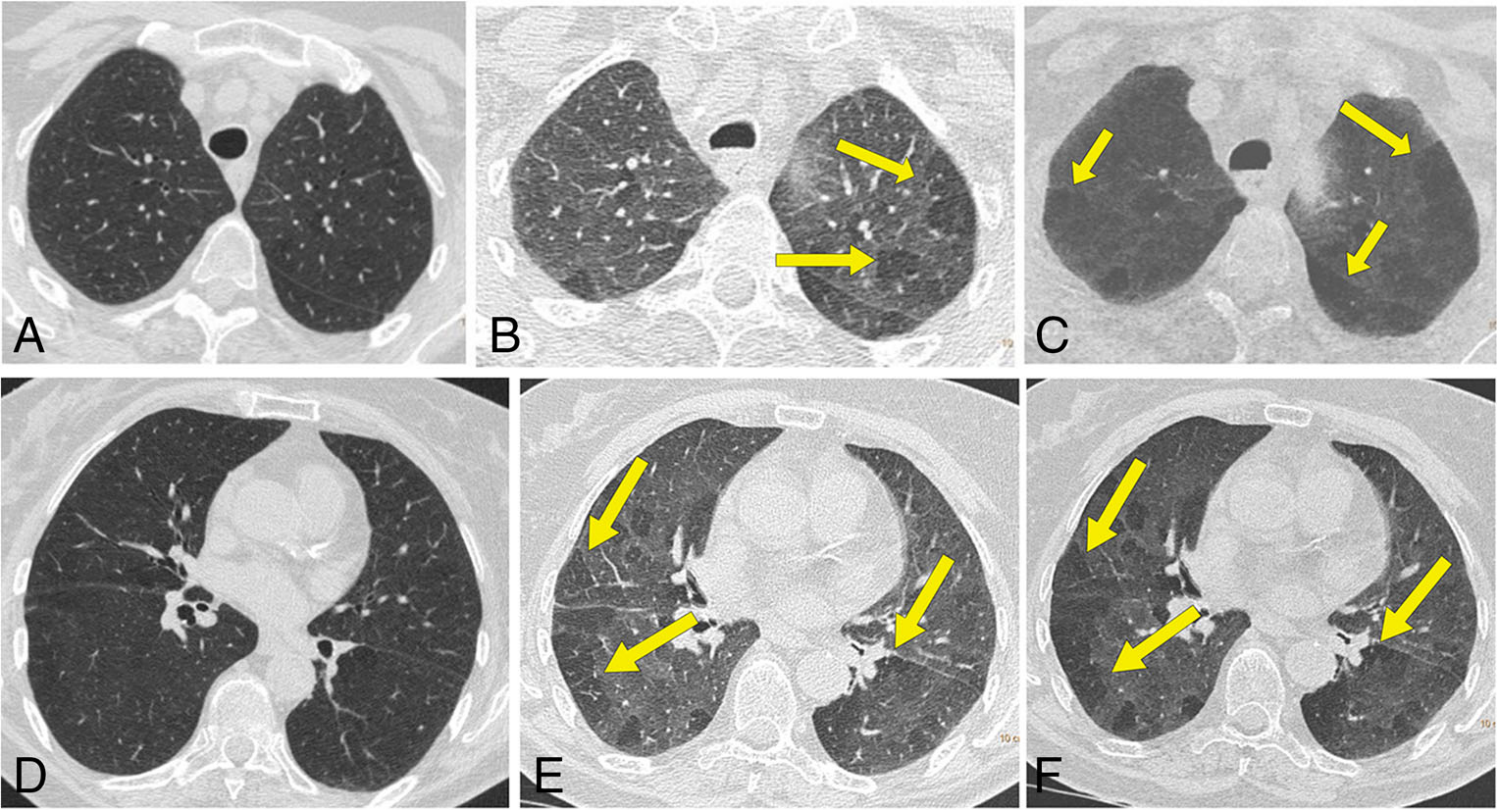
A 67-year-old nonsmoking man who had COVID-19. Inspiratory CT and expiratory CT scans at follow-up examination, 50 days after dyspnea and shortness of breath. A Inspiratory CT scan shows no abnormalities. B Expiratory CT scan obtained at same level as A shows significant multifocal areas of low attenuation due to air trapping in both upper lobes (arrows). C MiniP reformatted image is useful to improve the degree of air trapping (arrows). D Inspiratory CT scan at the level of the lower lobes shows no abnormality. E Expiratory CT scan obtained at same level as D shows significant multifocal areas of low attenuation due to air trapping in both lower lobes (arrows). F MiniP reformatted image also shows bilateral air trapping (arrows)
